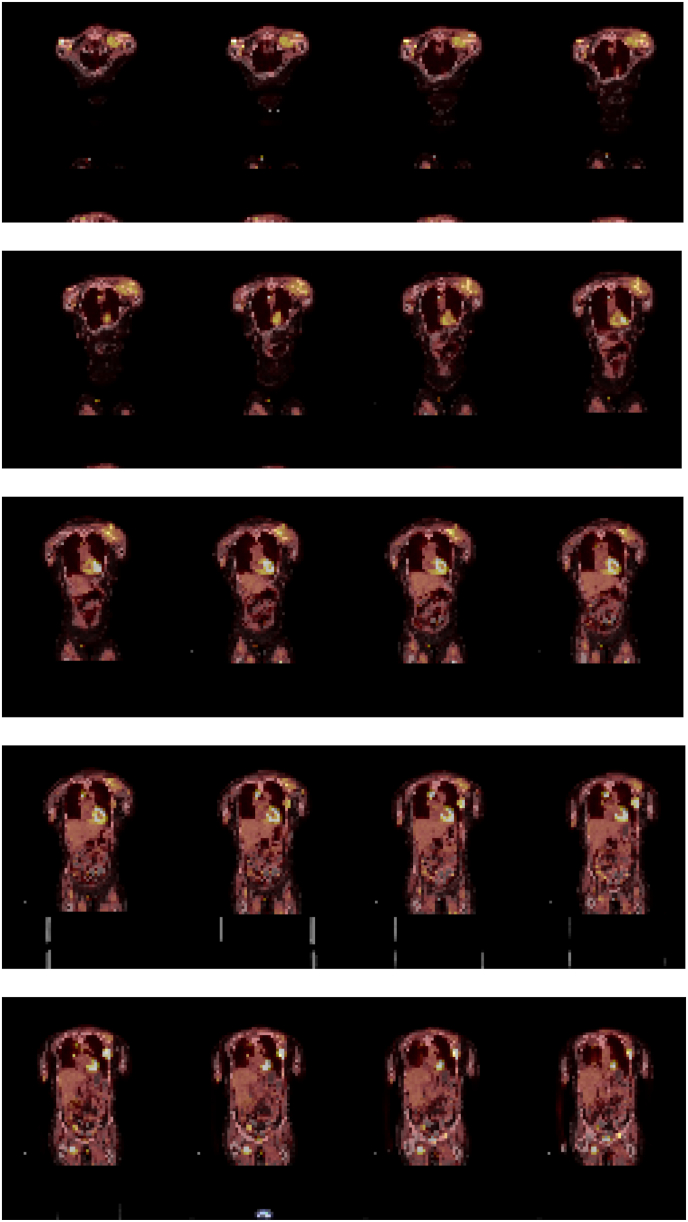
Patient 1 PET CT with increased tracer uptake at left shoulder.
